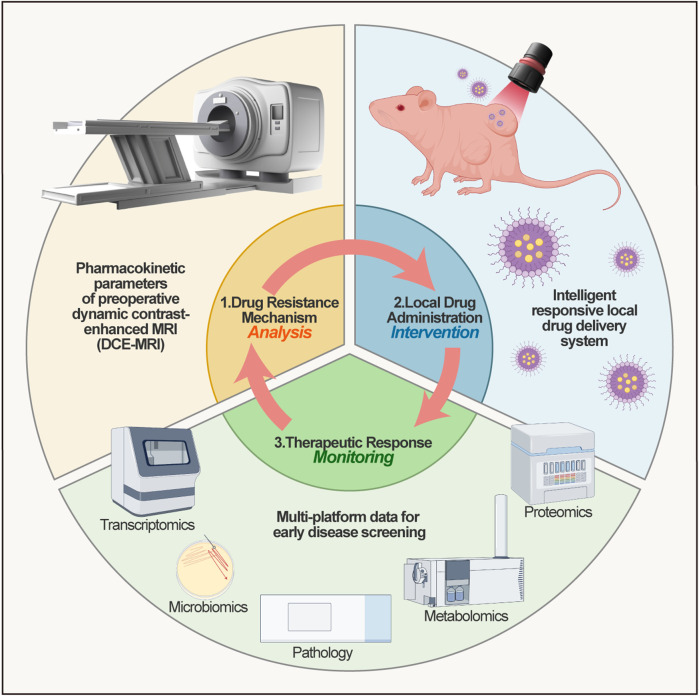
Precision Therapeutic Closed Loop Driven by Spatial Multi-Omics. The process begins with preoperative dynamic contrast-enhanced MRI (DCE-MRI) to characterize intratumoral pharmacokinetic heterogeneity, identifying resistant regions and guiding localized drug delivery. This is followed by intelligent drug administration intervention, utilizing a smart responsive localized drug delivery system to monitor therapeutic response. Finally, multi-platform data from transcriptomics, microbiomics, pathomics, proteomics, and metabolomics are integrated for real-time efficacy monitoring and algorithm iterative updates, forming a continuously optimized precision therapeutic closed loop. Created by Figdraw.
